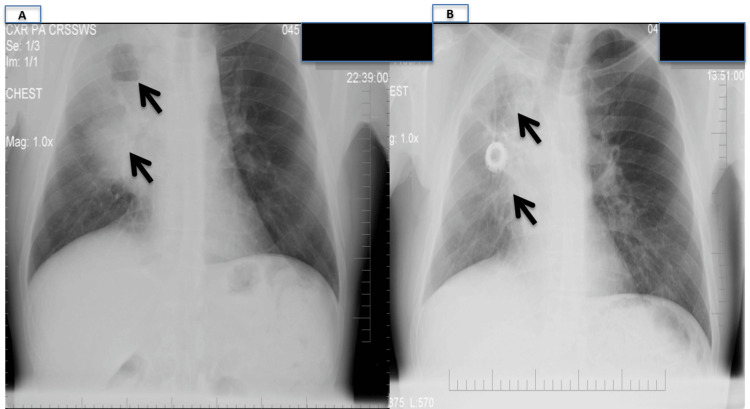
Chest X-rays (A) before and (B) after treatment was started and completed (black arrows).
